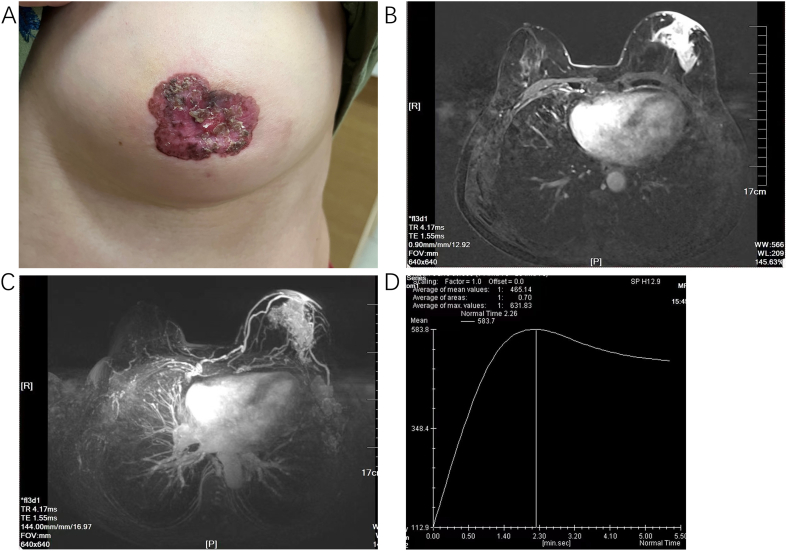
Appearance of breasts and magnetic resonance images from the patient at initial diagnosis. (A) Appearance of breasts of this patient. (B) T2 fat saturation imaging revealed high signal at the rear of the left nipple, about 51 × 60 × 36 mm in size. (C) Enhanced magnetic resonance imaging. (D) The signal-intensity time curves of tumors.
